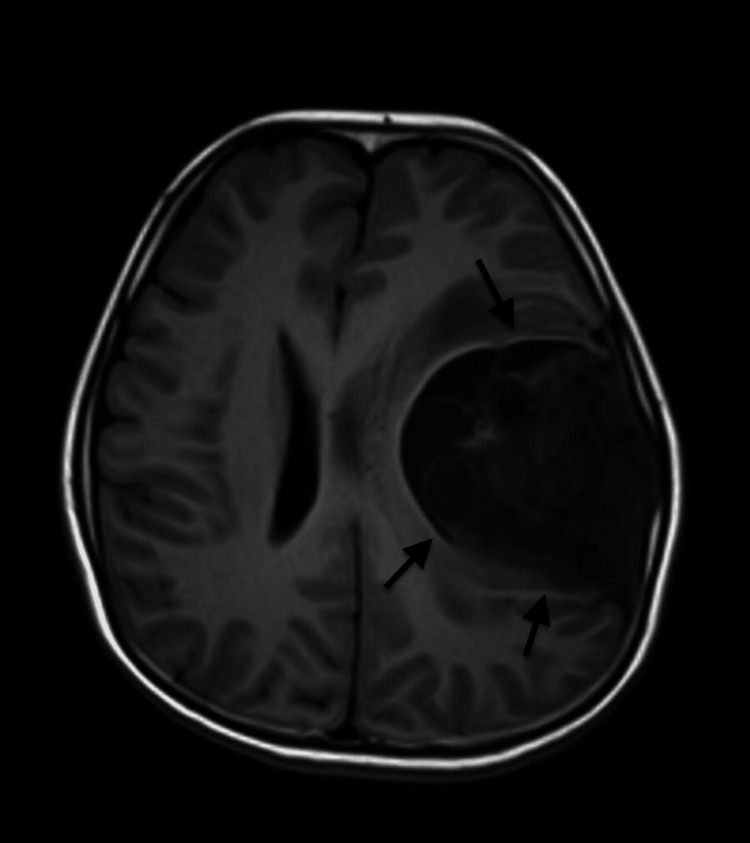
Preoperative magnetic resonance imaging scan in the T1 axial view Arrows are pointing toward the tumor.
